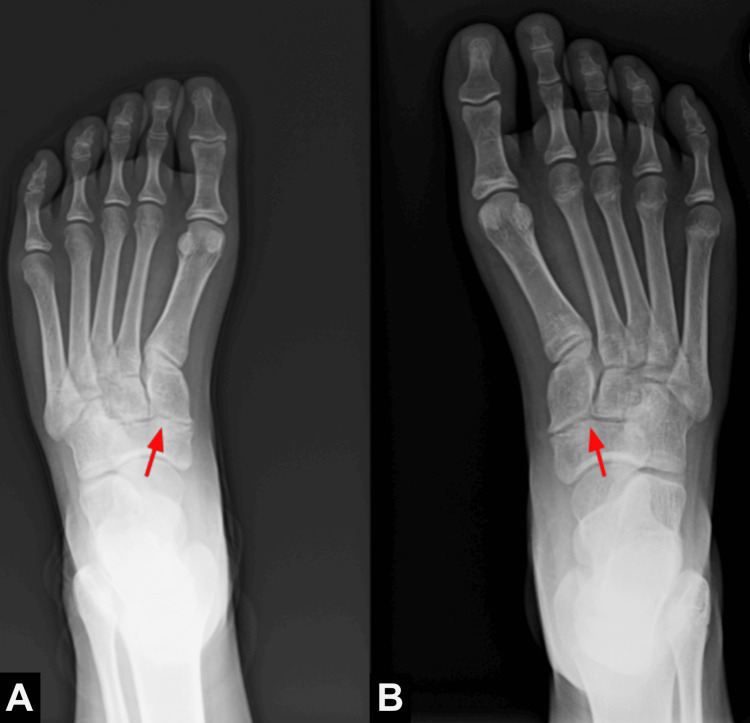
Frontal radiographs of the (A) left and (B) right feet demonstrating bilateral spurring vs. osteophytosis at the medial margins of the naviculocuneiform joints.
